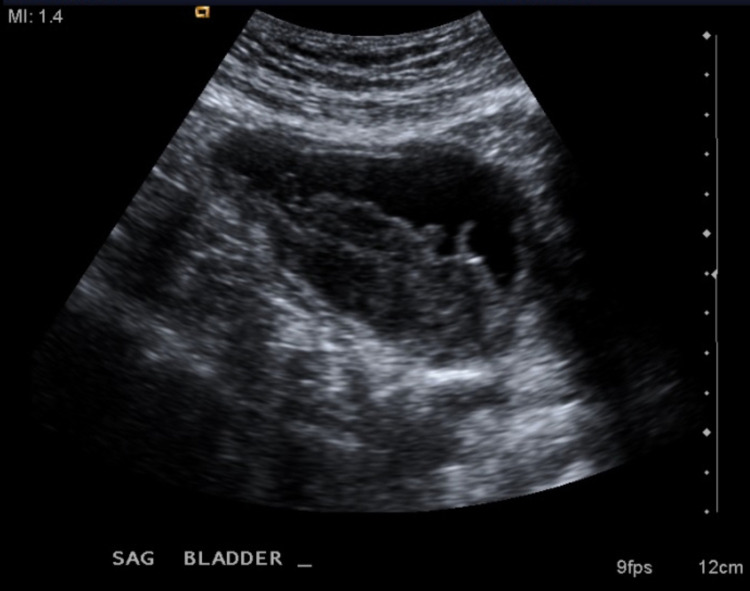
Ultrasound Findings This sagittal view of the bladder reveals a mass measuring 8.4 × 3.1 × 5.4 cm^3^ with observance of blood flow.
